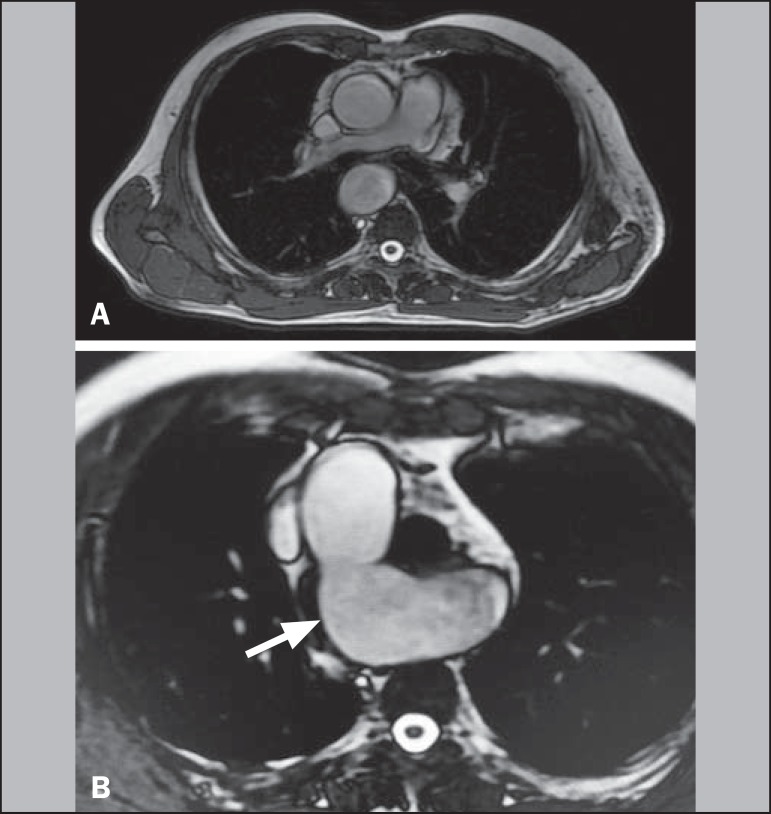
A,B: Axial T2-weighted spin-echo magnetic resonance imaging
